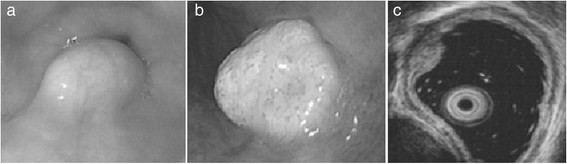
Endoscopic and endoscopic ultrasound (EUS) image of different esophageal granular cell tumors (GCTs). (a) Small yellowish peanut-like half protrusion under the smooth overlying mucosa. (b) A submucosal knurl with a small tuberculum. (c) EUS view of esophageal GCT (mildly heterogeneous solid pattern originating from the muscularis mucosa layer).
